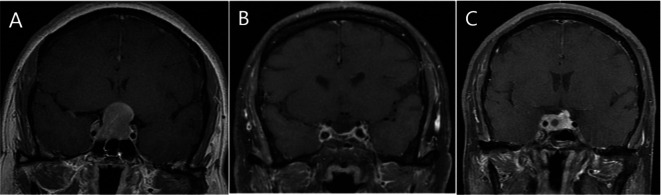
Preoperative T1-enhanced MRI image of the patient (A) shows a lesion in the saddle area, with the surgical pathology indicating a pituitary adenoma. The 4-month postoperative re-examination MRI of the saddle area (B) shows no obvious tumor residue, while the 3-year postoperative re-examination MRI (C) shows a mass in the saddle area, suggesting tumor recurrence.
